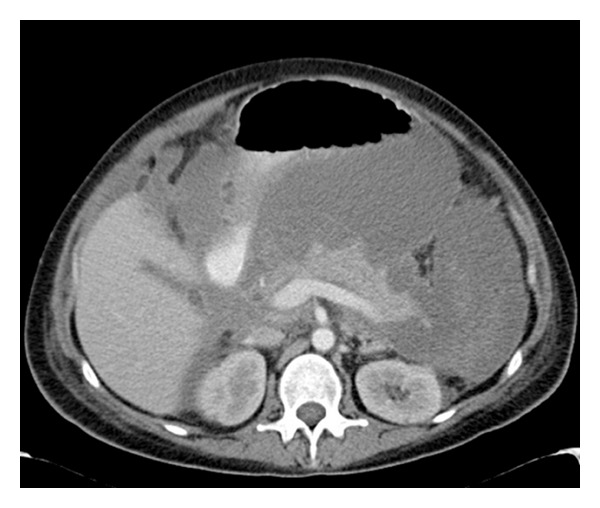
Axial contrast-enhanced CT abdomen 14 days after-admission showing gross ascites and pancreatic parenchymal enhancement.
